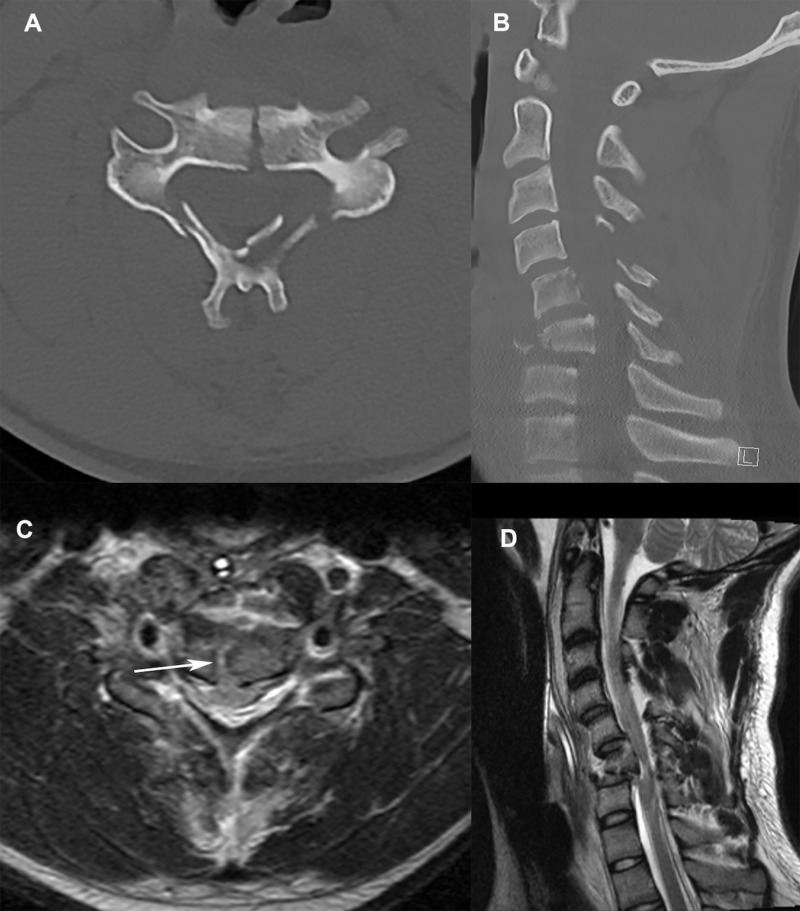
Cervical spine imaging at presentation A. Axial CT of the cervical spine at the C6 level showing the vertebral body fracture as well as the laminar fractures; B. Sagittal CT of the cervical spine shows focal kyphotic deformity at the C6 level associated with the burst fracture with significant canal stenosis; C. Axial MRI of the cervical spine shows herniation of the spinal cord into the vertebral body (arrow); D. Sagittal MRI of the cervical spine showing retropulsion of the C6 vertebral body into the spinal canal with significant spinal cord compression. CT: computed tomography; MRI: magnetic resonance imaging
